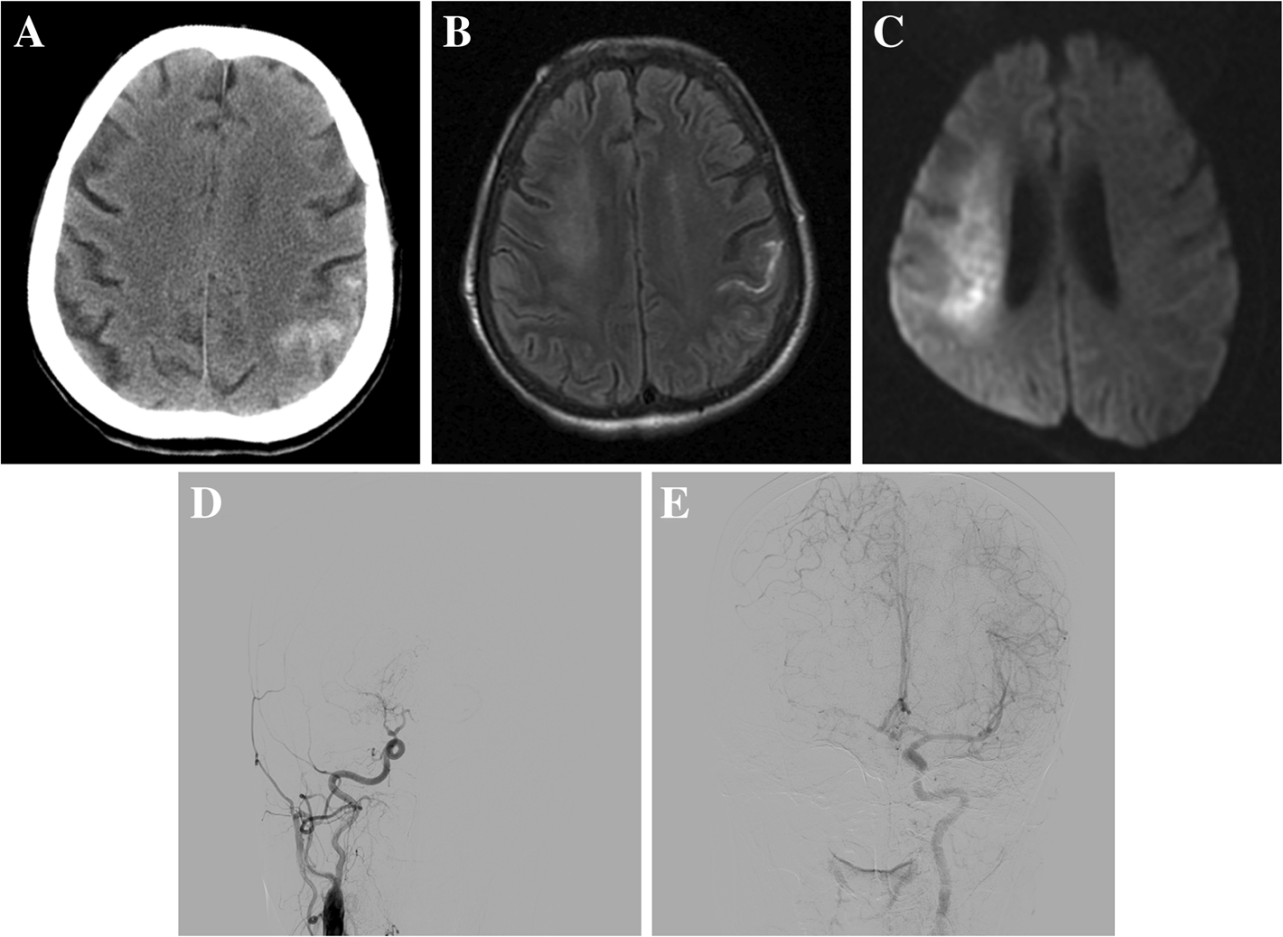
A 56-year-old female patient with cSAH due to cardioembolic stroke. a CT demonstrated a left parietal SAH. b T2-weighted fluid-attenuated inversion recovery MRI showed high-signal intensity on the left parietal convexity. c Diffusion-weighted imaging demonstrated acute ischemia in the right middle cerebral artery region. Digital subtraction angiography showed occlusion of the right ICA (d), and compensatory flow from the ipsilateral anterior cerebral artery via the leptomeningeal artery (e)
